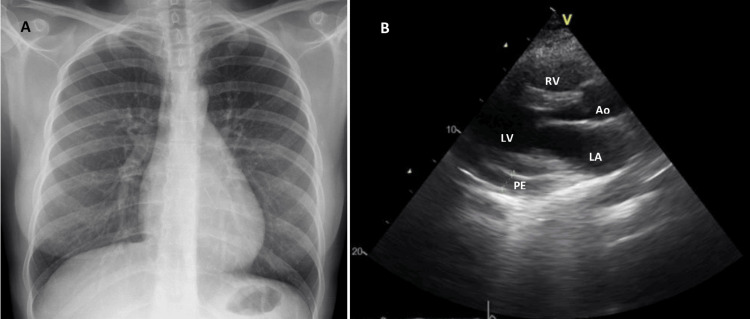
Follow-up chest radiography (A) and echocardiogram (B) revealing minor pericardial effusion PE: pericardial effusion; LA: left atrium; LV: left ventricle; Ao: aortic valve; RV: right ventricle
